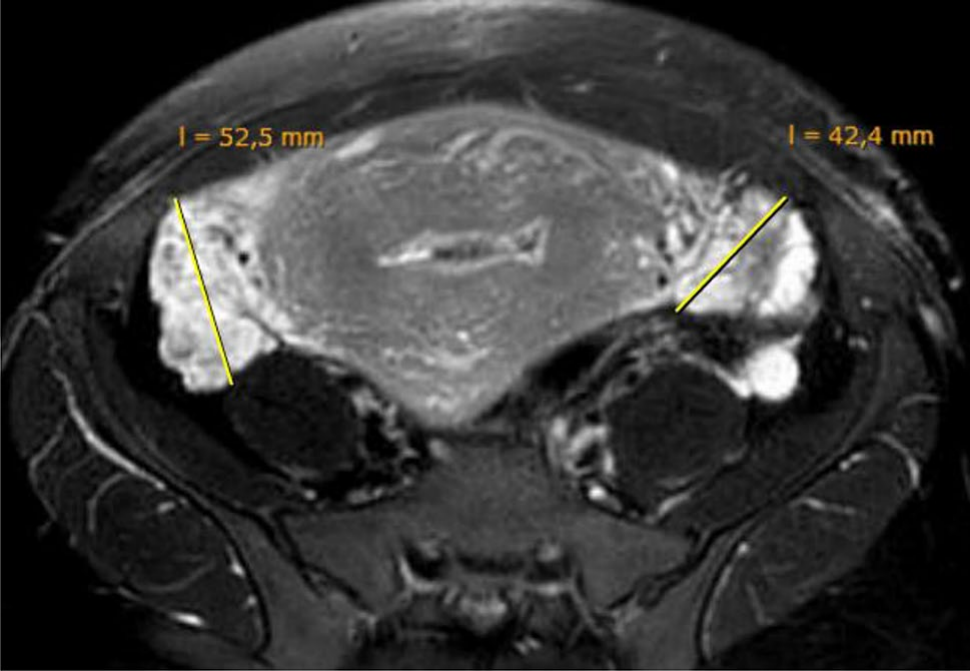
Pelvic magnetic resonance imaging of the same patient, 2 days after delivery. Apart from prominent ovaries (right ovary: 52.5 mm diameter; left ovary: 42.4 mm diameter) no abnormalities were detected
